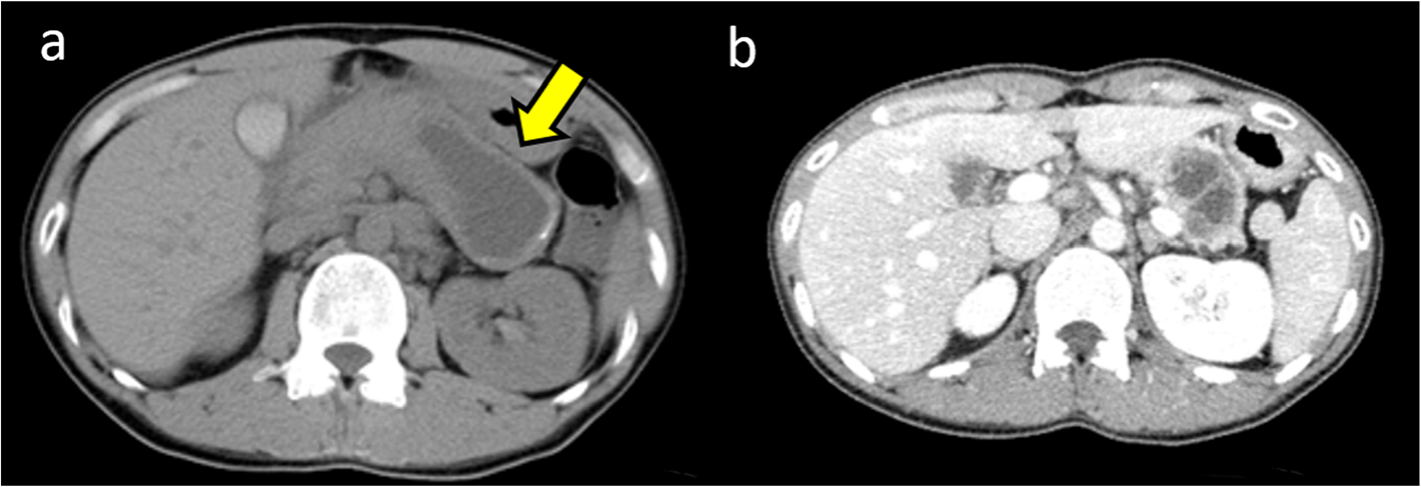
Computed tomography (CT) at onset revealed the presence of a cystic tumor measuring 60 mm in diameter in the pancreatic tail (a, arrow). Enhanced CT in our department also revealed a tumor in the pancreatic tail with an internal septal formation (b). There was no evidence of a nodular lesion in the tumor or ascites.
